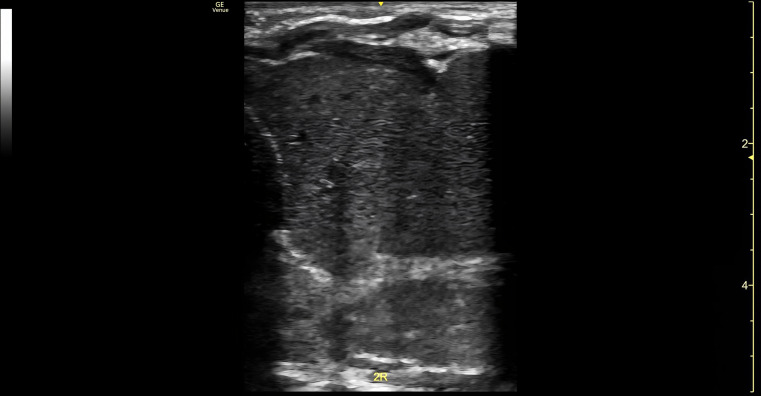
Point of care ultrasound (POCUS) of the right lung demonstrating homogenous echogenic structure within the right hemithorax consistent with liver parenchyma.
